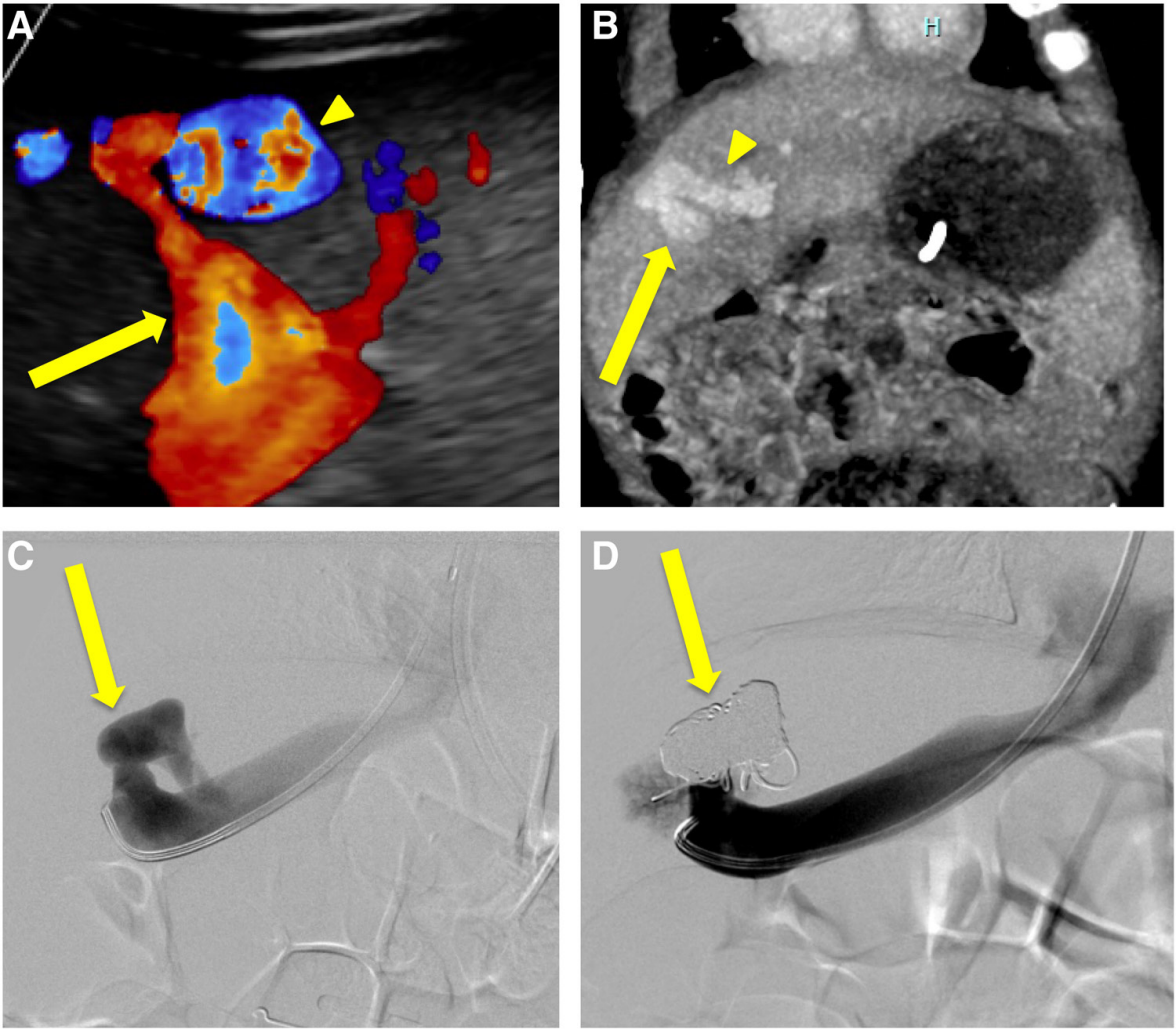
Imaging and embolization of the intrahepatic shunt. A and B) The patient’s ultrasound and CT showed an abnormal connection between the middle hepatic vein (arrow) and right portal vein (arrowhead), near the periphery of the liver. C and D) The shunt (arrow) was embolized from a transjugular approach using detachable coils. CT = computerized tomography.
